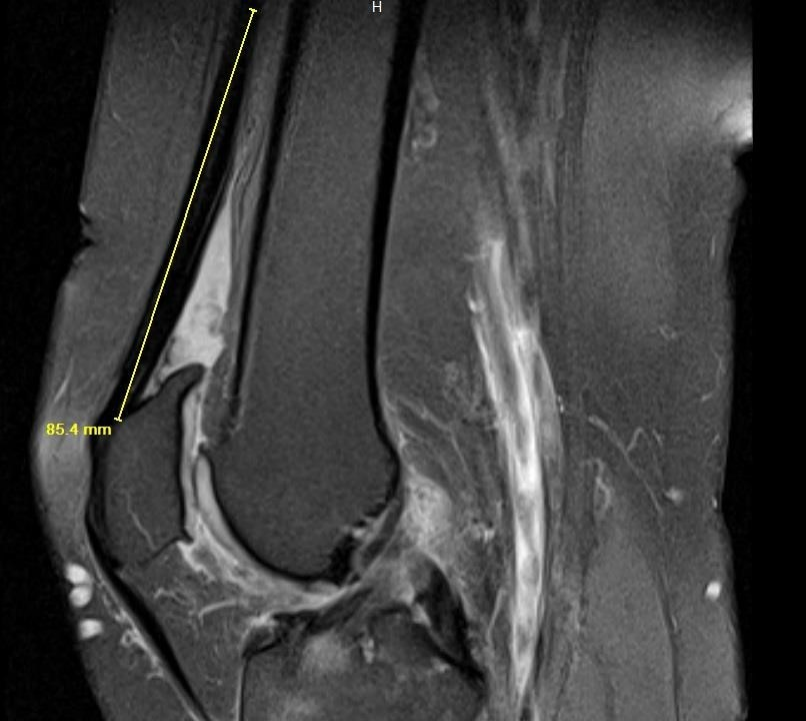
Midsagittal MRI image of the knee using the superior pole of the patella (distal endpoint) and musculotendinous junction of the rectus femoris muscle (proximal endpoint) as anatomic landmarks to measure the QT preoperatively.
